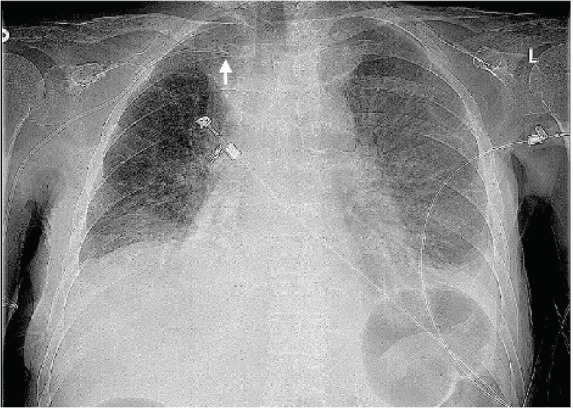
Radiograph identifying migrated, right-sided peripherally inserted central catheter with tip in proximal right subclavian vein (arrow) with bilateral pleural effusions.
